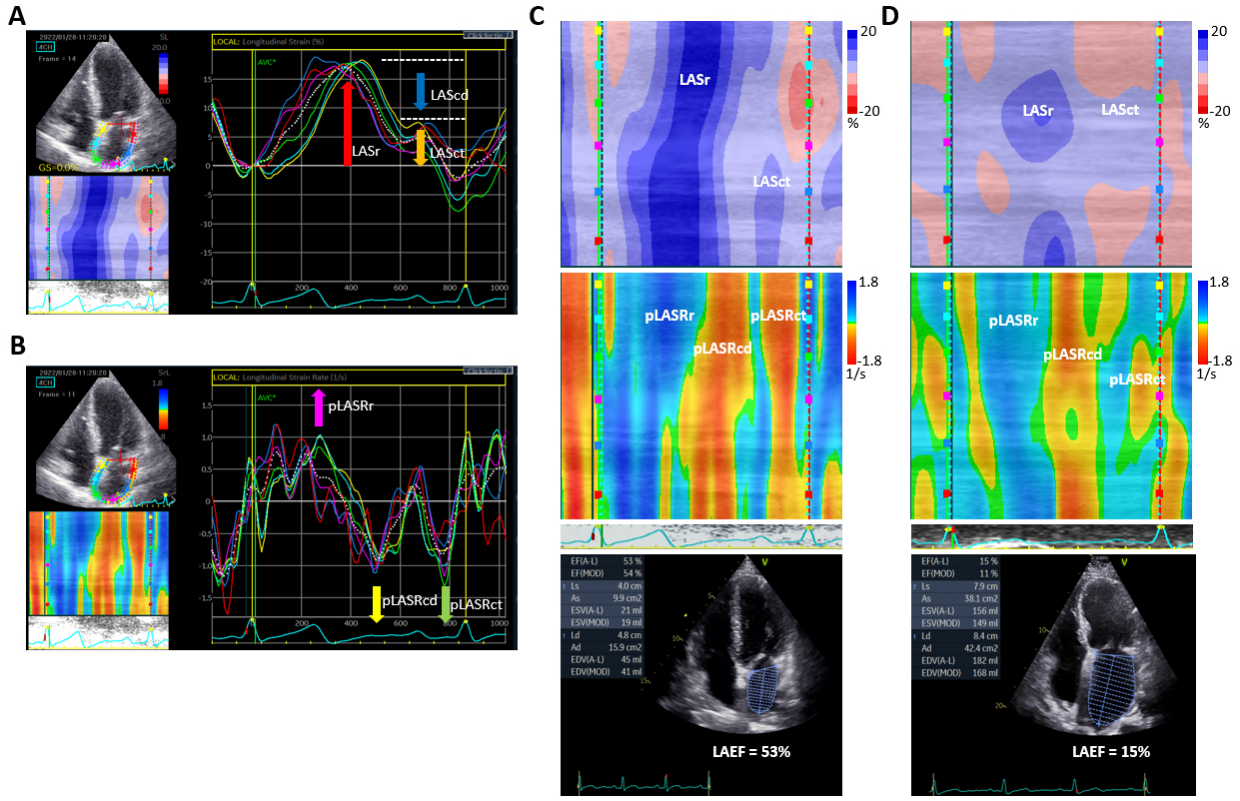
Transthoracic echocardiography in the apical four-chamber view
